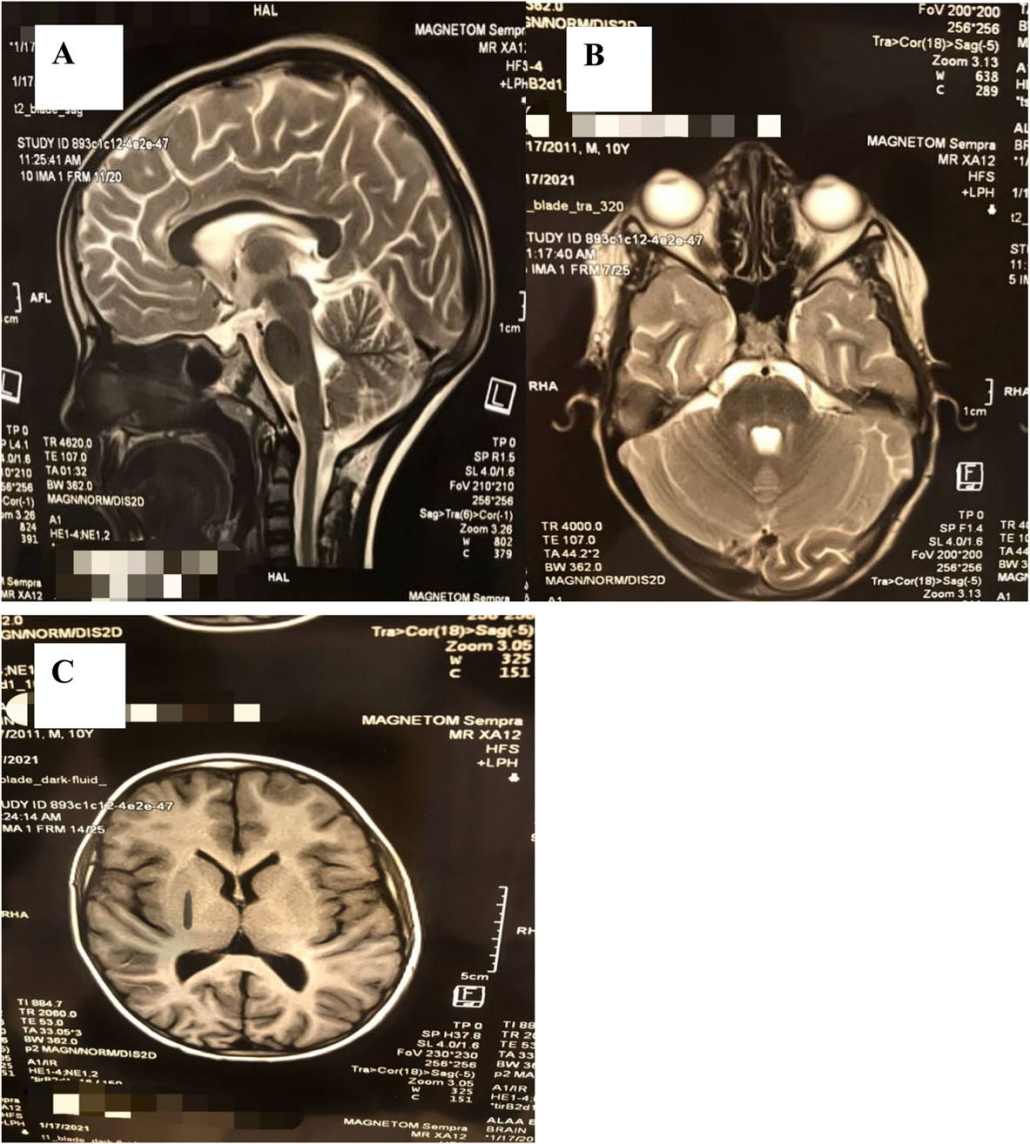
Brain MRI showed A normal sella turcica appearance, B normal perioptic disc space, and C normal size of ventricles
